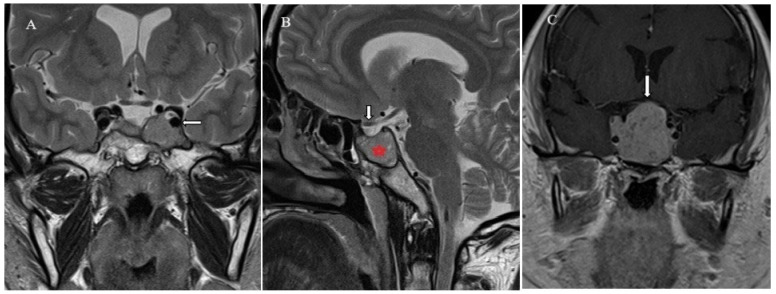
MRI images. (A) Coronal and (B) sagittal T2-weighted MR images show an isointense mass, which expands the left adenohypophysis (asterisk) and encases the carotid arteries (long arrow) but does not compress the optic chiasm (short arrow). (C) Coronal contrast-enhanced T1-weighted MR image shows mild homogeneous enhancement of the giant pituitary macroadenoma with elevation and compression of the optic chiasm (arrow indicates the area of optic chiasm).
